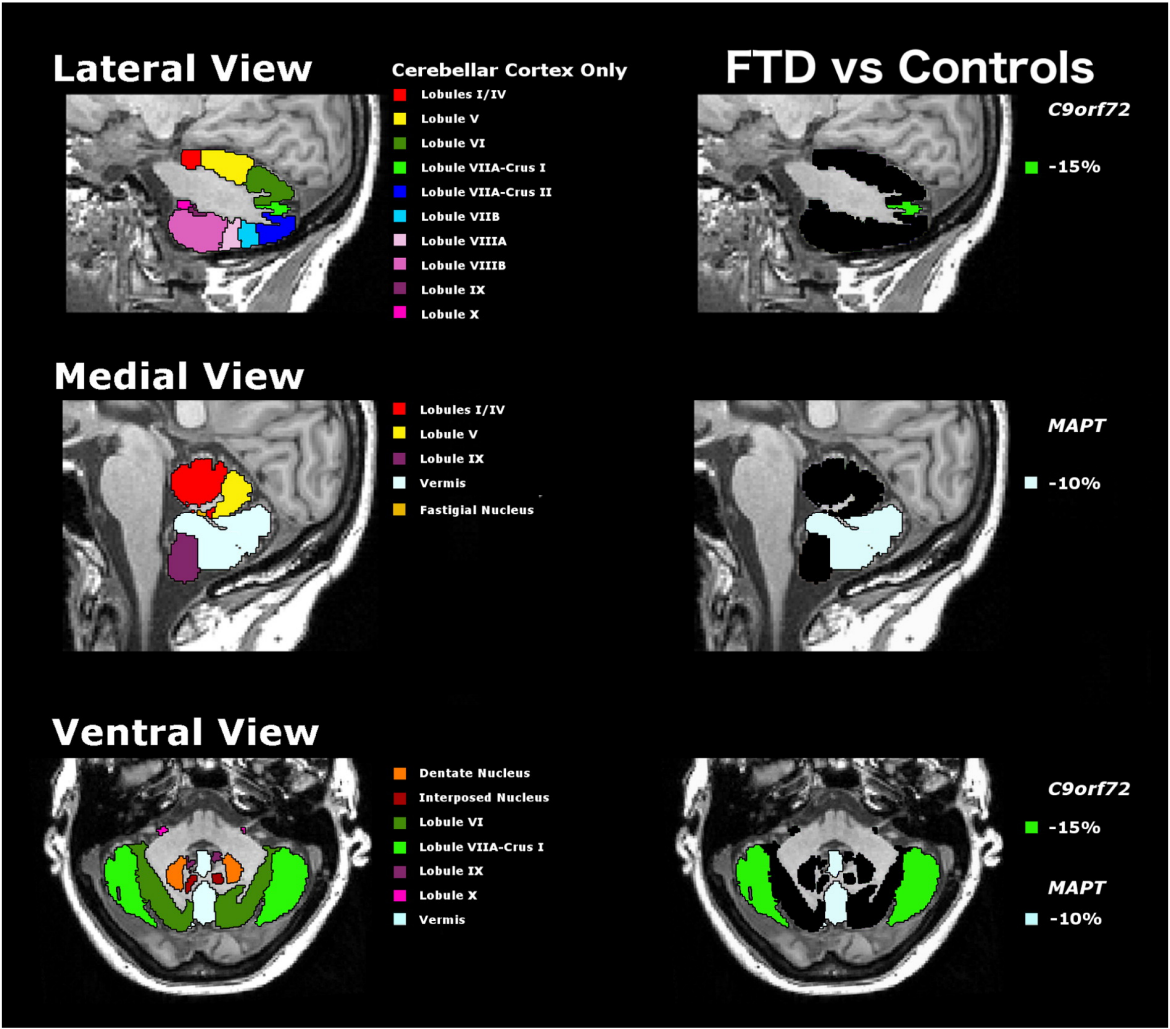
Segmentation of the cerebellar lobules and deep nuclei (left side) mapped on a T1-weighted 3T MR image of a control subject. On the right panel significant volumetric differences in FTD are mapped on the same MR image.
